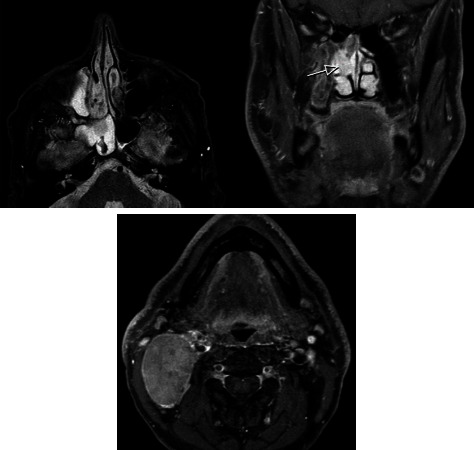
Axial STIR, coronal, and axial postcontrast T1-weighted with fat saturation MR images of patient no. 3 with a T3N2bM0 tumor located in the right nasal cavity and sphenoid sinus. On the axial image, the primary tumor has an intermediate signal intensity. Note: due to obstruction by the tumor, the right maxillary sinus and the sphenoid sinus are filled with retained secretions displaying high signal intensity. The coronal image shows the enhancing tumor in the right nasal cavity just medial to the middle turbinate (arrow). Axial postcontrast image lower in the neck with a markedly enlarged lymph node in level II (fine needle aspiration cytology: TCS lymph node metastasis).
